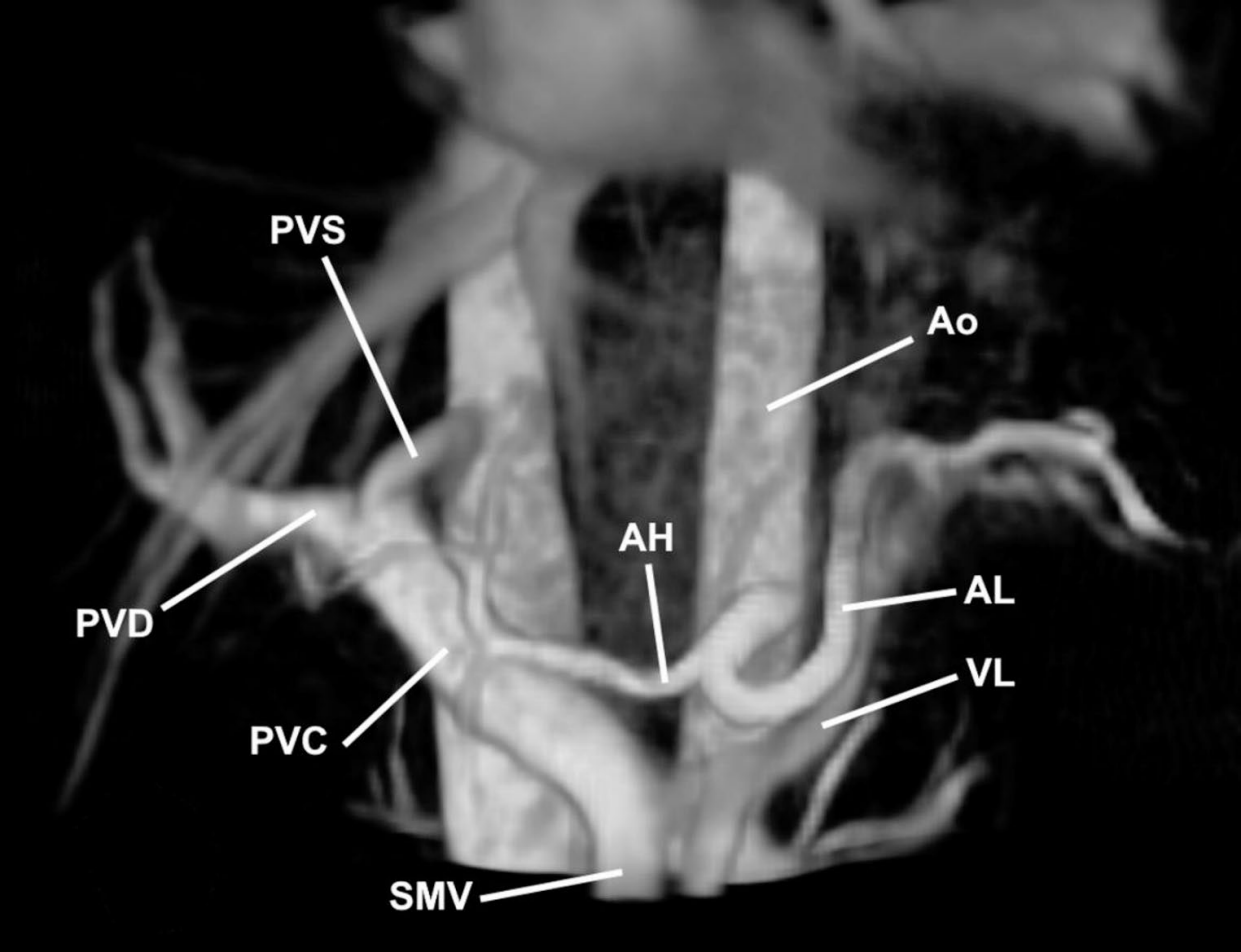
Overview of splanchnic blood vessels measured with abdominal 4D Flow MRI in a healthy volunteer (21 years old, male). *Ao* Abdominal Aorta, *AL* Splenic Artery (Arteria Lienalis), *AH* Hepatic Artery (Arteria Hepatica), *VL* Splenic Vein (Vena Lienalis), *VMS* Superior Mesenteric Vein (Vena Mesenterica Superior), *VPC* Common Portal Vein (Vena Porta Communis), *VPS* Left Portal Vein Branch (Vena Porta Sinistra), *VPD* Right Portal Vein Branch (Vena Porta Dextra)
